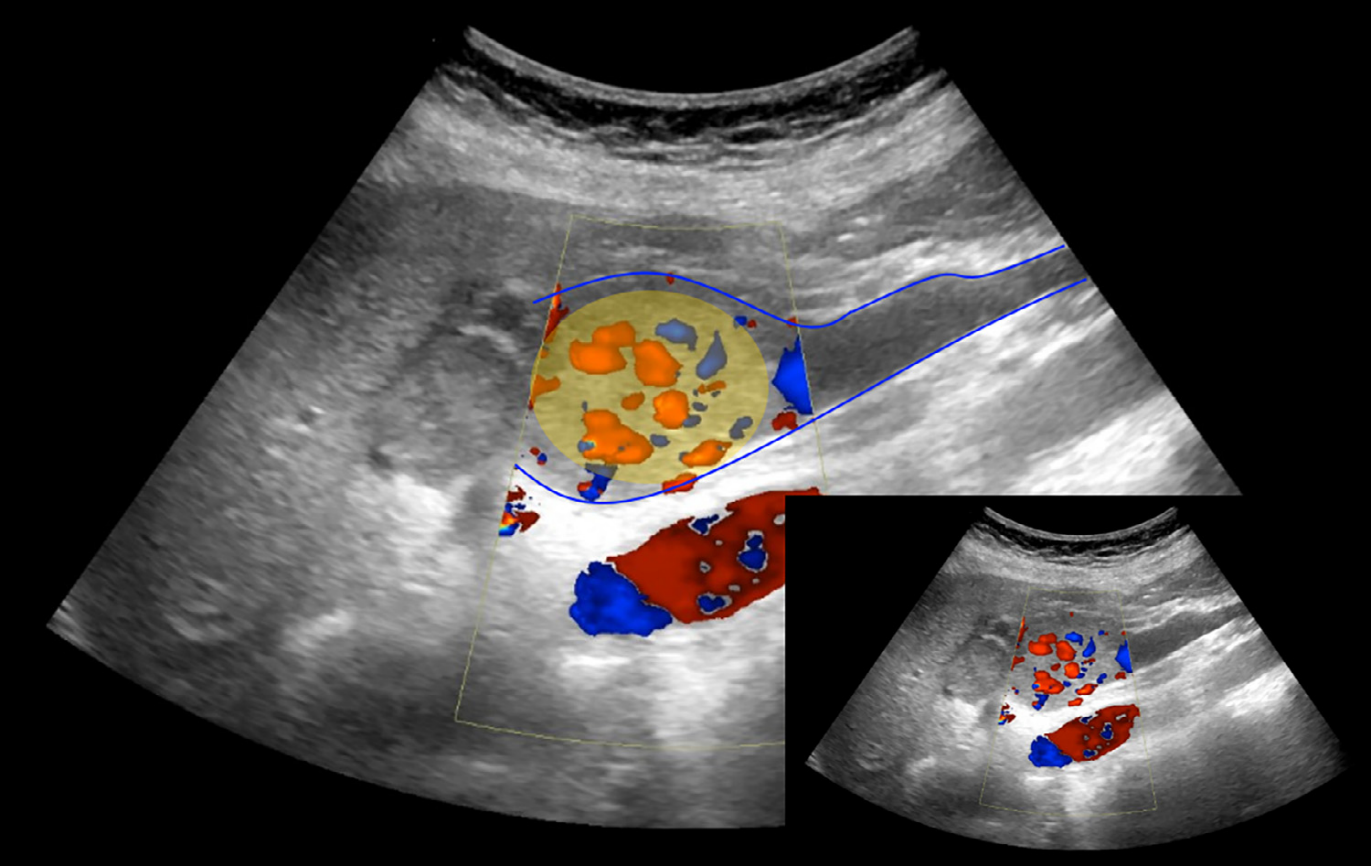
Ultrasound picture showing existence of vascularity inside the tumoral thrombosis (yellow).
